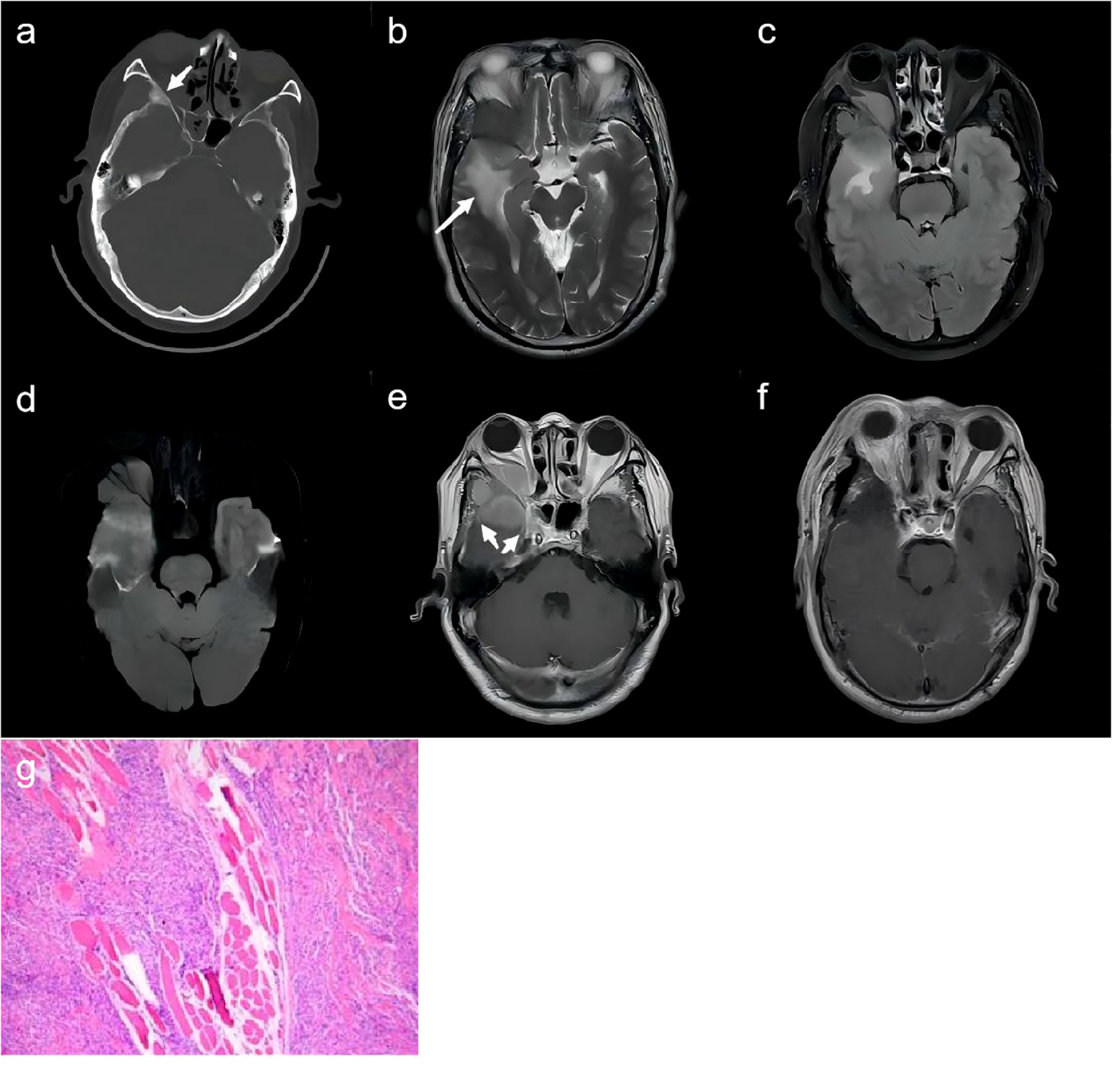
A 56-year-old man. (A) CT scan with bone window demonstrates the spheno-orbital osteocondensing lesion (white arrow). (B–D) MR images depict the right spheno-fronto-temporo-orbital mass with edema (white arrows) of the surrounding brain parenchyma which showed hyperintensity on T2WI (B), T2-weighted fluid-attenuated inversion recovery (C), and diffusion sequence (D). (E) The lesion shows homogeneous enhancement with involved meningeal enhancement (white arrows) on postcontrast T1-weighted. (F) The post-operative enhanced MRI scans revealed patchy enhancement within the region that was previously occupied by the lesion. (G) Pathological examination revealed meningothelial meningioma (CNS WHO grade I) with epithelioid tumor cells.
